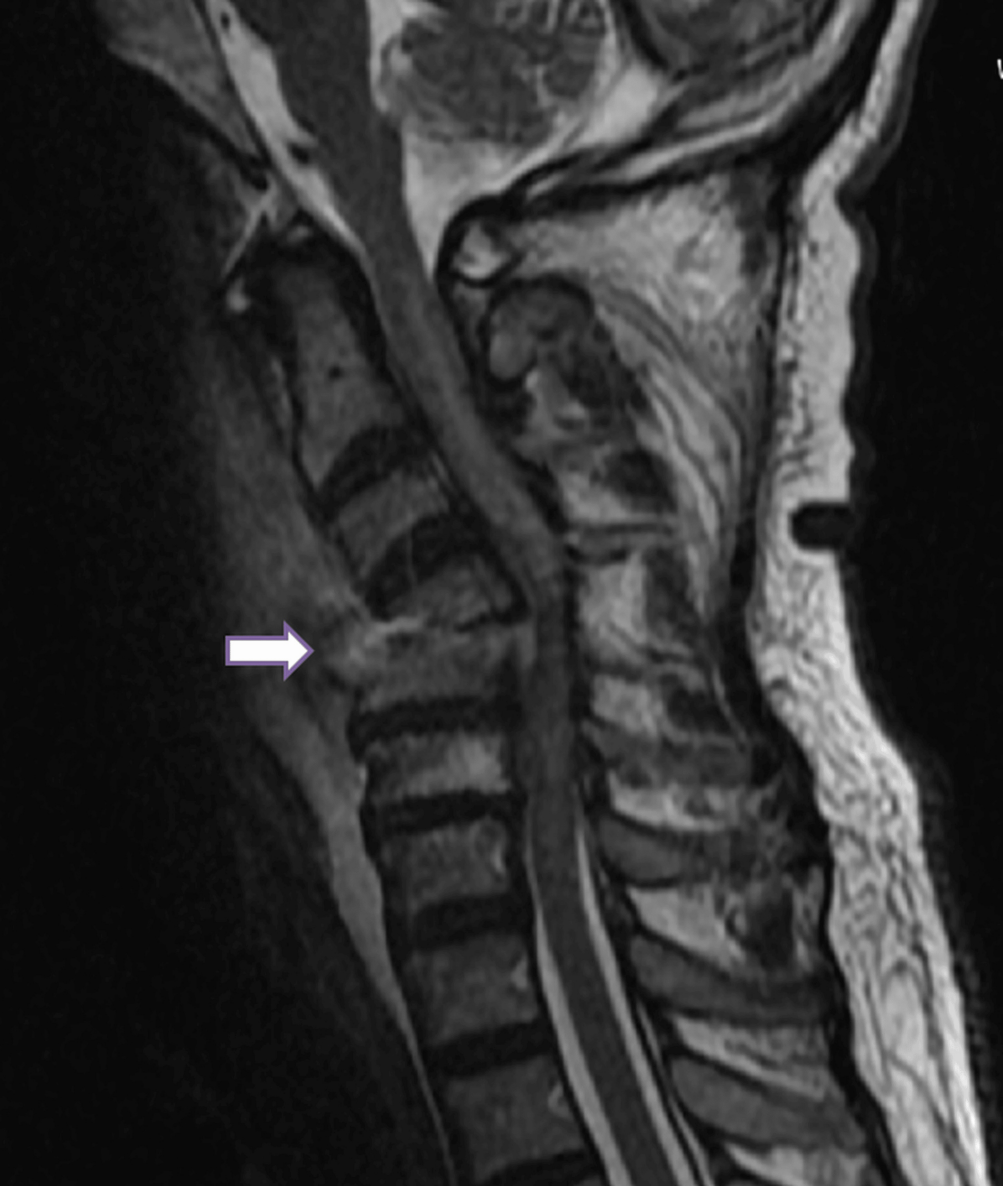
Sagittal T2-weighted MRI of the cervical spine. Collapse of the C5 vertebral body with heterogeneous marrow signal, posterior retropulsion, and severe spinal cord compression at the C4–C5 level (white arrow). Intramedullary T2 hyperintensity within the cord indicates edema.
